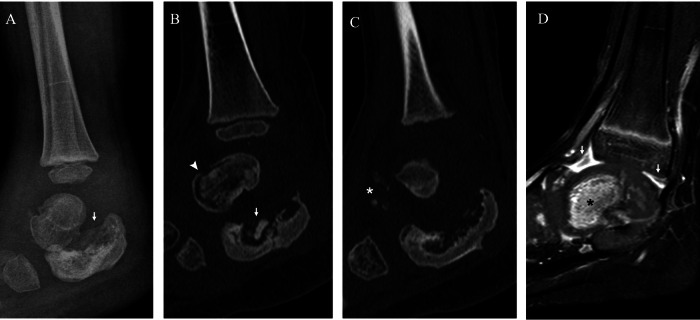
Neuropathic arthropathy of the right ankle in a 3-year-old boy with hereditary sensory and autonomic neuropathy type IV. Lateral radiograph at injury (A) shows sclerotic and lytic changes of the calcaneus with bone collapse (arrow). Sagittal CT (B, C) confirms fragmentation of the calcaneus (arrow) and shows subcortical fracture of the talus (arrowhead) and intra-articular loose bodies (white asterisk). Sagittal T1-weighted post-gadolinium MRI (D) shows bone marrow enhancement of the talus (black asterisk) and diffuse synovial hyperenhancement (arrows)
